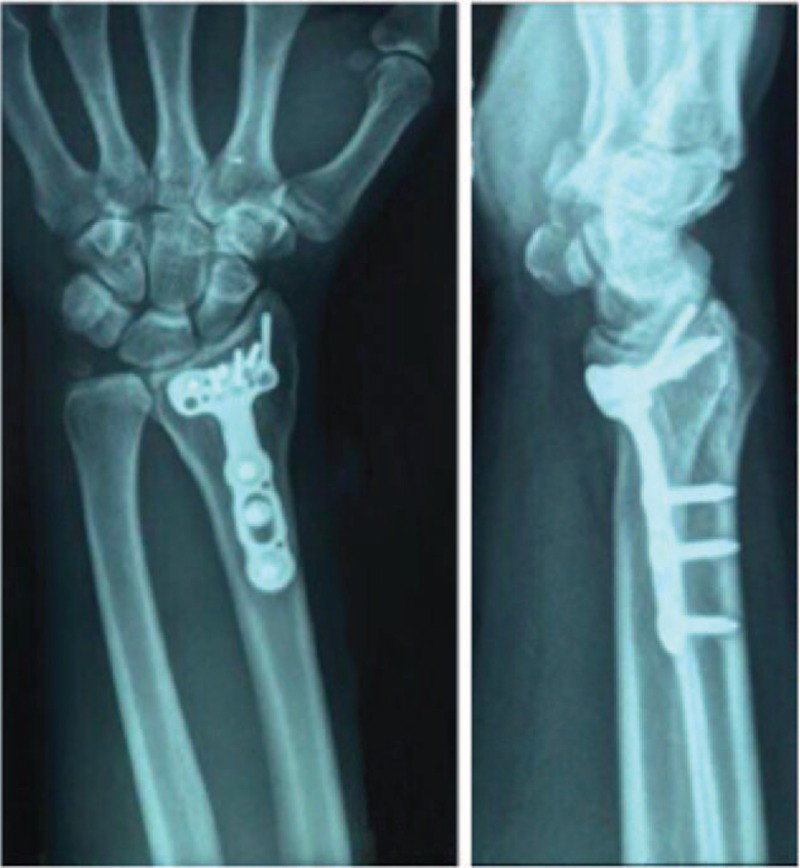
Preoperative x-ray of right radius plate removal surgery.
